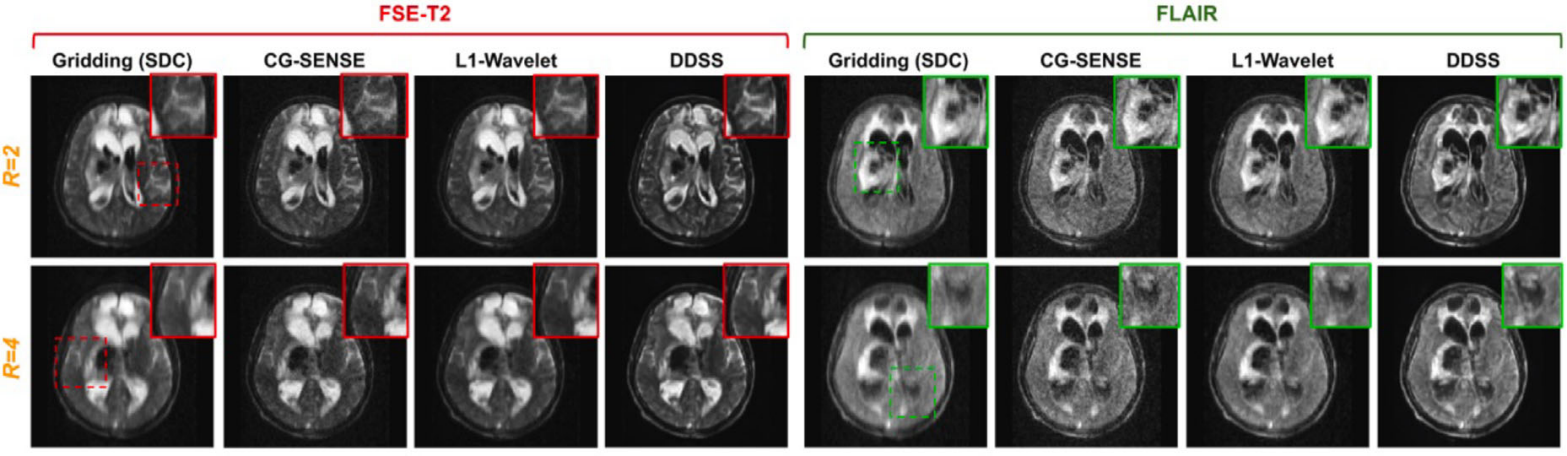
Image reused from Zhou et al.^35^ Qualitative comparisons of FSE‐$T_{2}$ weighted and FLAIR reconstructions from real data acquired from a low‐field (64 mT) MRI scanner. The subject was diagnosed with a hemorrhagic stroke with associated midline shift. The proposed self‐supervised reconstruction (DDSS) is compared to gridding,^67^ L1‐wavelet,^68^ and CG‐SENSE^69^ reconstructions.
